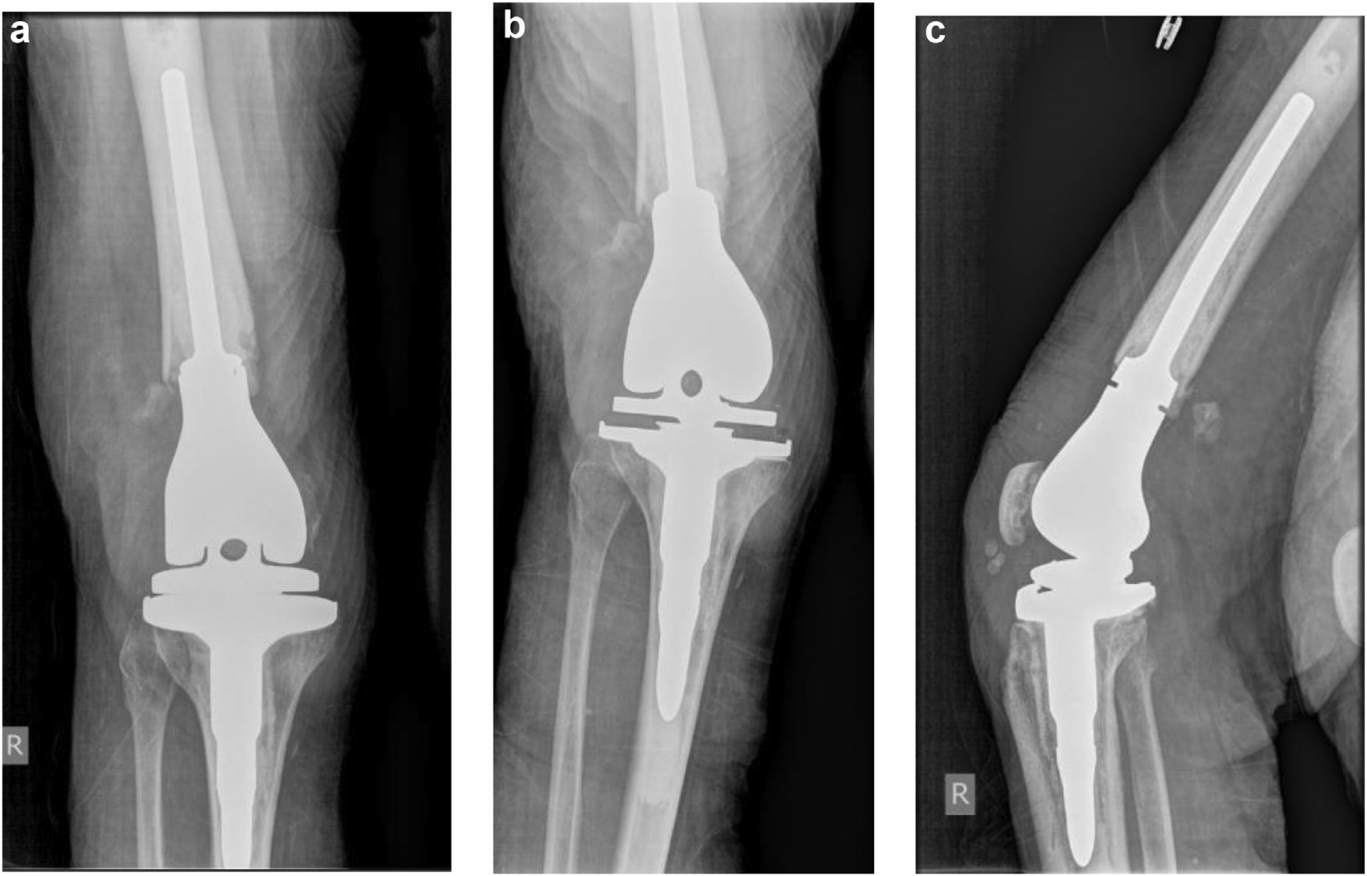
Anteroposterior (a and b) and lateral (c) 1-year postoperative radiographs demonstrating cemented distal femoral replacement with maintenance of alignment, rotation, and fixation. Insall-Salvati ratio of 1.22.
